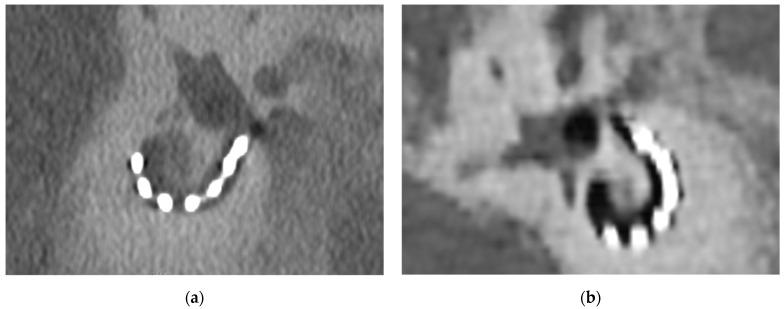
Comparison of the visibility of an implanted CI and the surrounding temporal bone. (a) PCD-CT image of CI following implantation surgery at 160 kV; (b) EID-CT image of CI following implantation surgery at 160 kV. The metal-to-bone contrast is better for the PCD-CT images.
